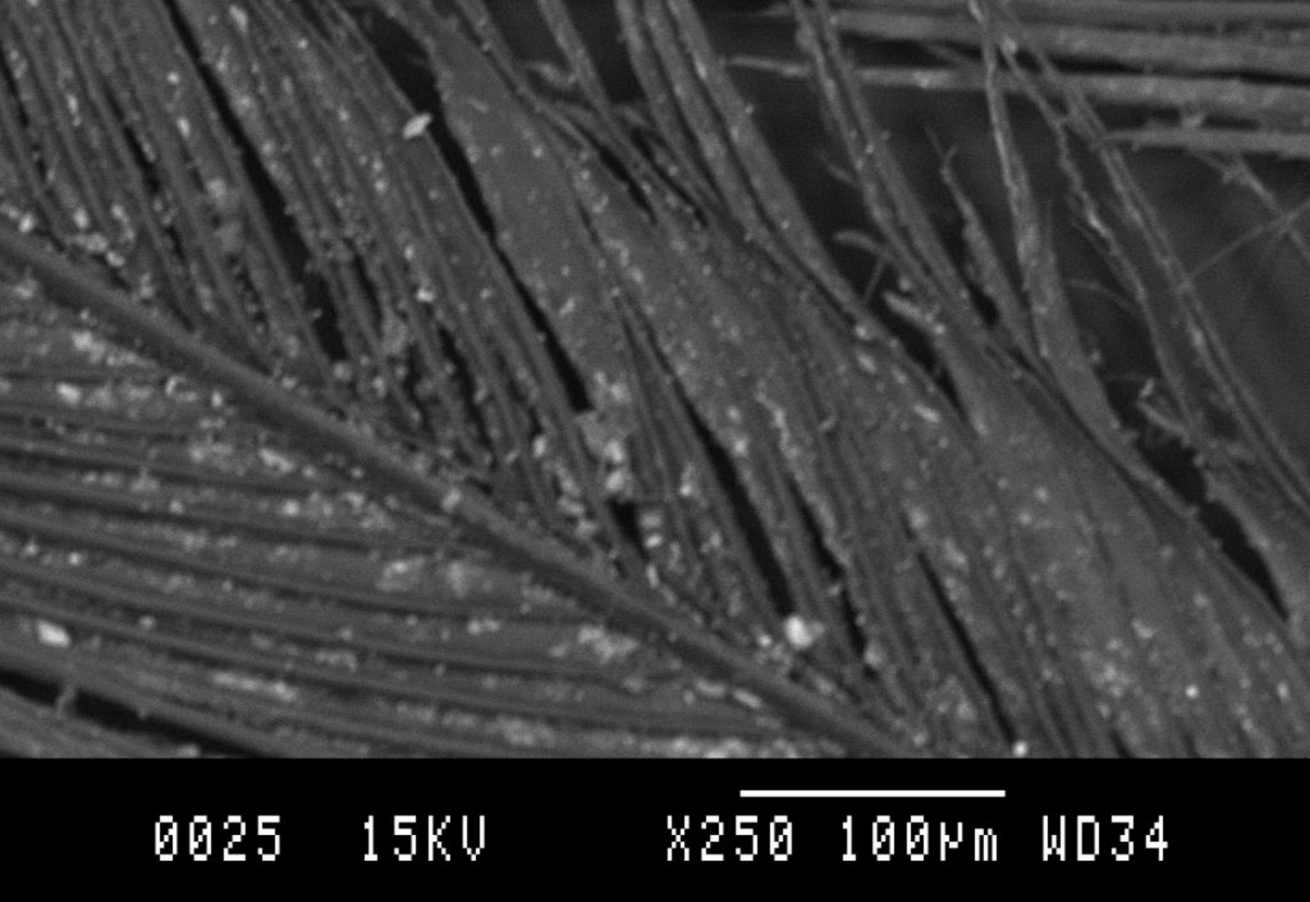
Detail of a X-ray picture carried out on an orange-coloured Bearded Vulture feather.
